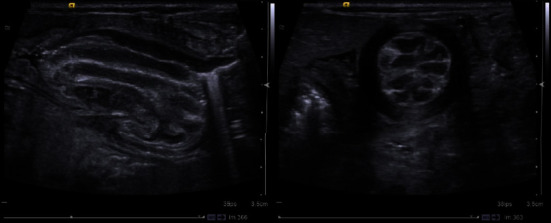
Longitudinal and transverse ultrasound views of the pylorus displaying intussusception.
